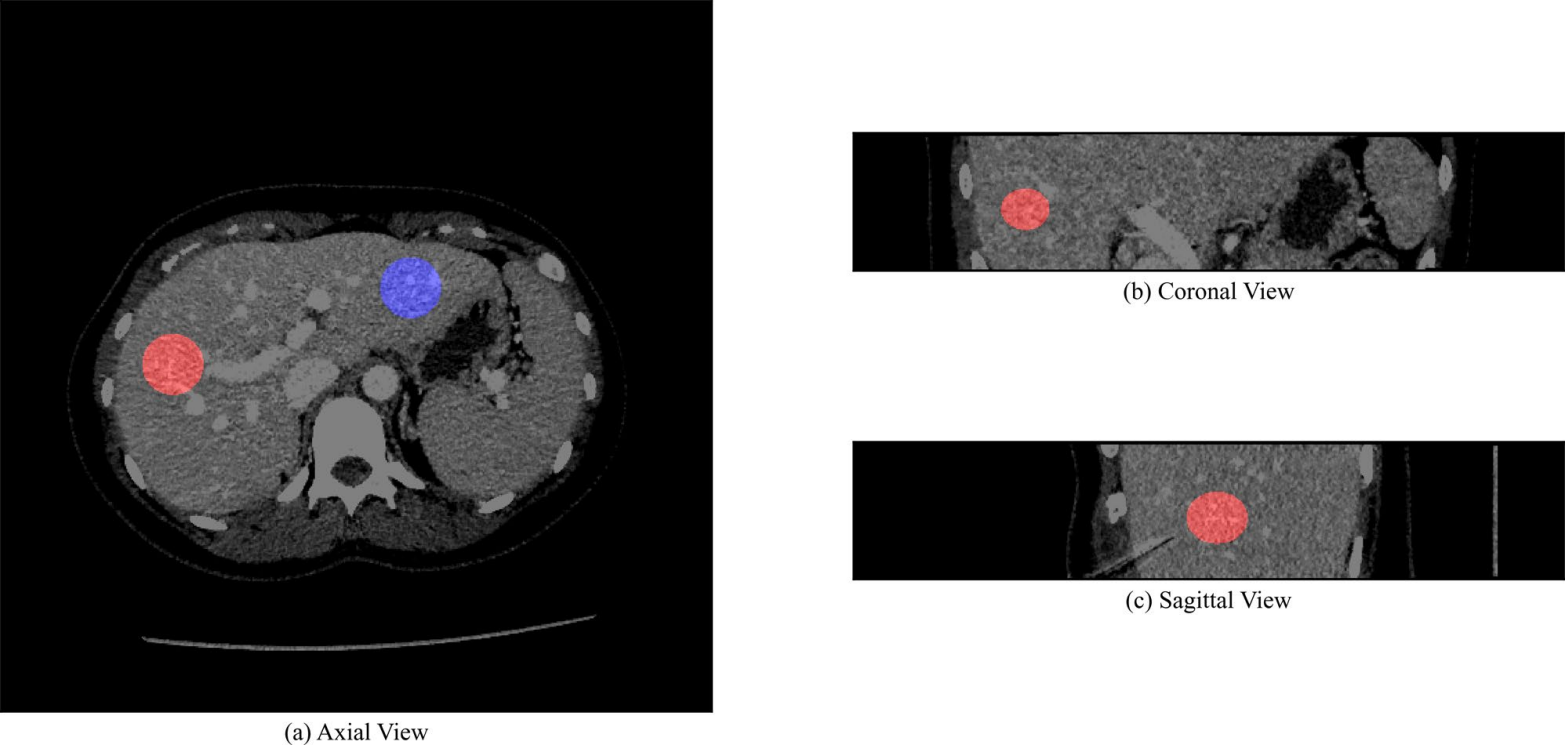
Example CT image with axial (a), coronal (b), and sagittal (c) views. The biopsy-based ROI is visualized in red and the non-biopsy ROI is visualized in blue. The biopsy needle is visible in the sagittal view
